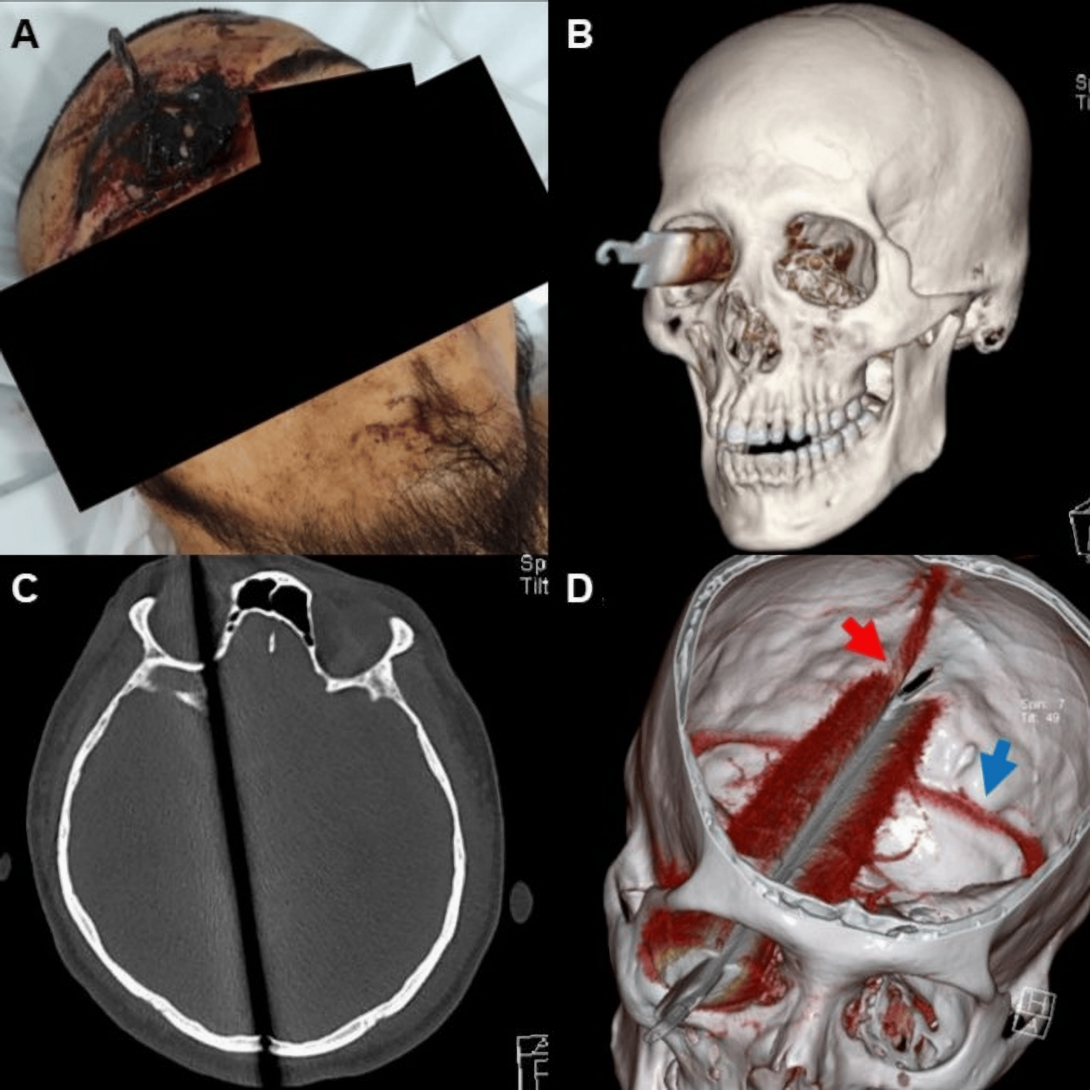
(A) Physical examination of the transorbital trauma. (B) Computer tomography (CT) 3D preoperative reconstruction where the entry of the cutting object into the orbit is appreciable. (C) Bone window of the CT where the trajectory of the knife from the right orbit to the left parasagittal line, passing through the entire occipital bone, is observed. (D) CT 3D reconstruction where it is observed that the sharp object passes too close to the superior sagittal sinus (red arrow) and the transverse sinus (blue arrow).
